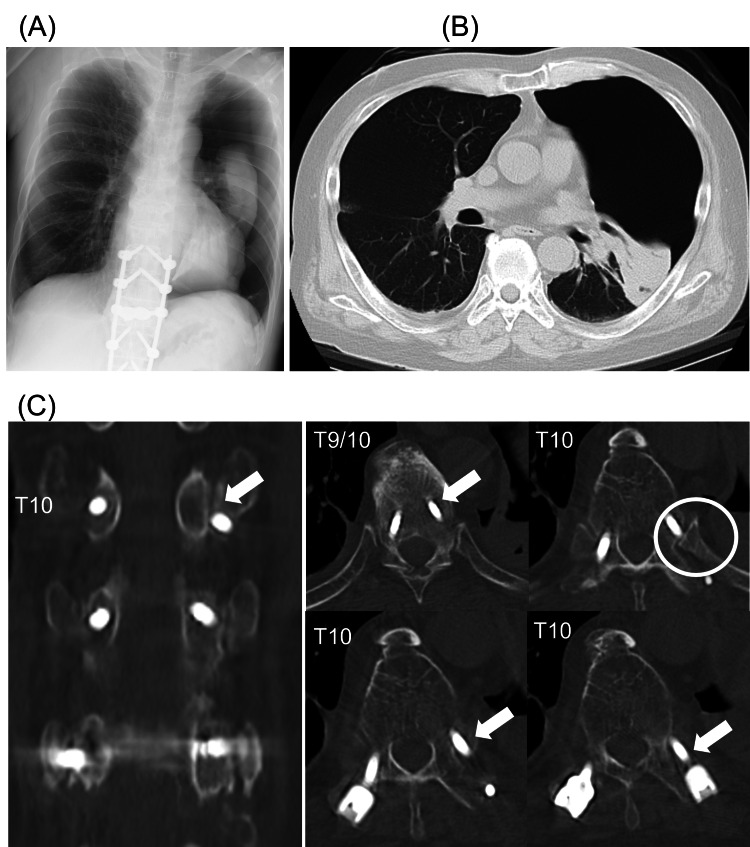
Radiological findings two days after surgery. Pneumothorax on the left side was observed in the chest x-ray (A) and the chest CT (B). Postoperative spine CT (C) showed that the left screw at T10 was placed lateral to the pedicle but caught vertebral body (arrow). The rib head at left T10 displaced to the anterior compared with the right side (circle). CT: computed tomography
